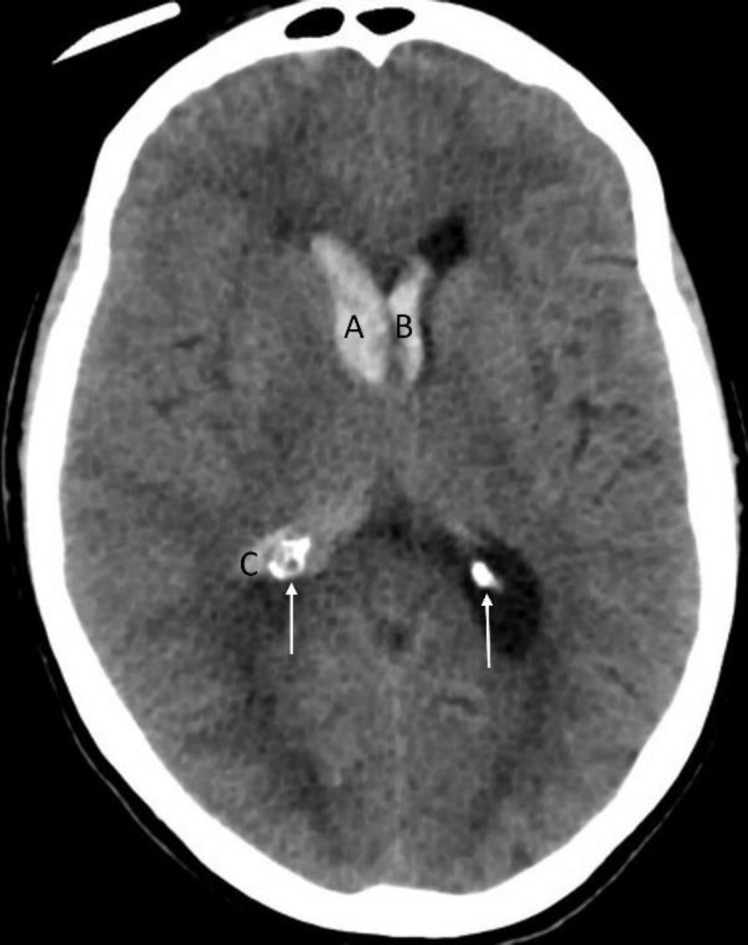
Non-contrast axial CT brain of a 78 years-old female with an intracerebral bleed showing intraventricular extension of bleed in the lateral ventricles (A-C). Note difference in the density of an acute bleed with that of calcification (arrows) of the choroid plexus. It is important to appreciate that, unlike acute bleed, density of calcification parallels that of the calvarium
